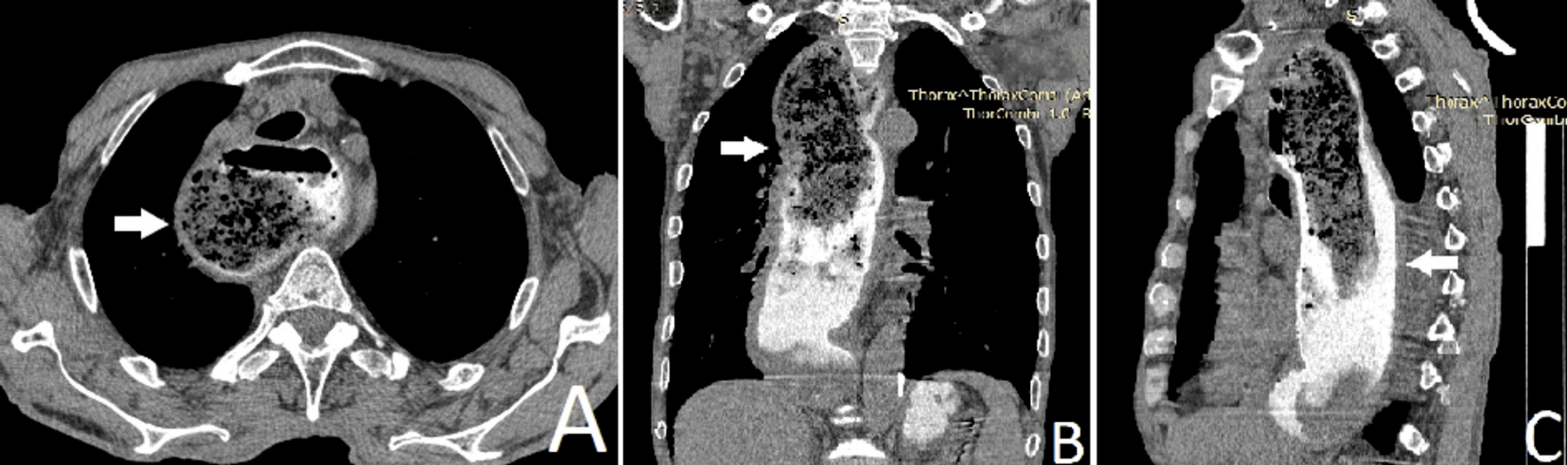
Аxial (A), coronary (B), and sagittal (C) CT views of the thoracic esophagus (the arrows show the dilated and tortuous esophagus) CT: computed tomography
